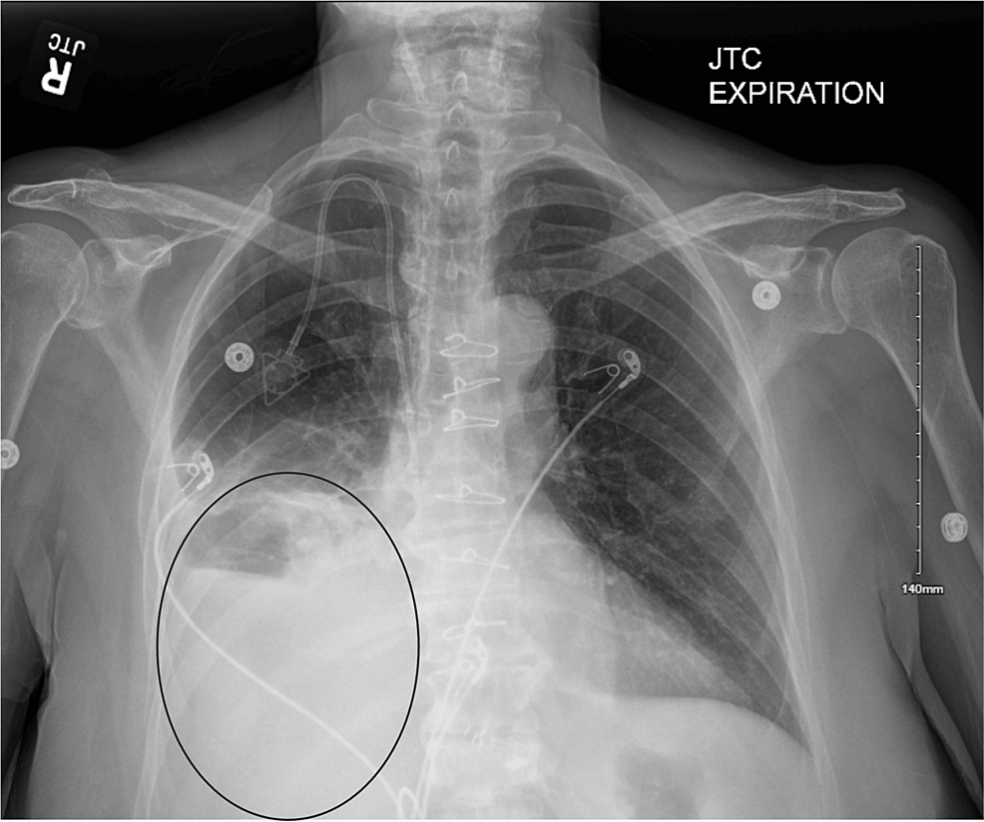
Chest X-Ray Displaying Right-Sided Pleural Effusion The encircled area demonstrates opacification in the right middle and lower lung lobes. There is also blunting of the right costophrenic angle.
